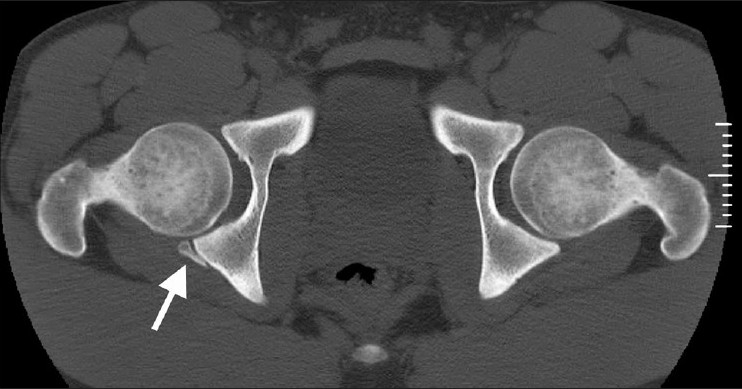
CT three months after injury shows a posterior lip right acetabular fracture with bridging bone at the posterior margin of the fragment
